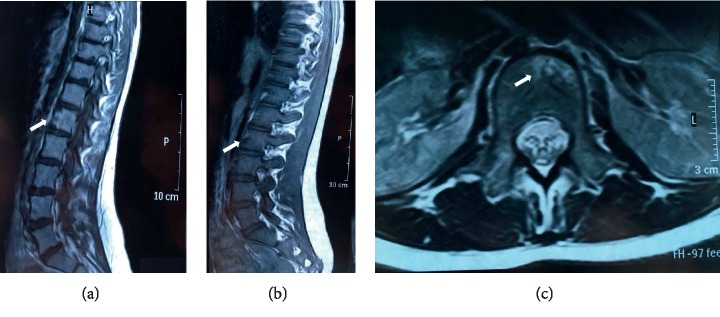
MRI of the dorsolumbar spinal cord. (a) Sagittal plane of the lumbar spinal cord, T1-weighted sequence with gadolinium contrast, and irregular increase of the signal on the front of vertebra L1 (arrow). (b) T2-weighted sequence, lack of recruitment in the same region (arrow). (c) Axial plane of vertebra L1, T1-weighted sequence with gadolinium contrast, and increase of the signal in the anterior third of the vertebral body (arrow) and conus medullaris.
